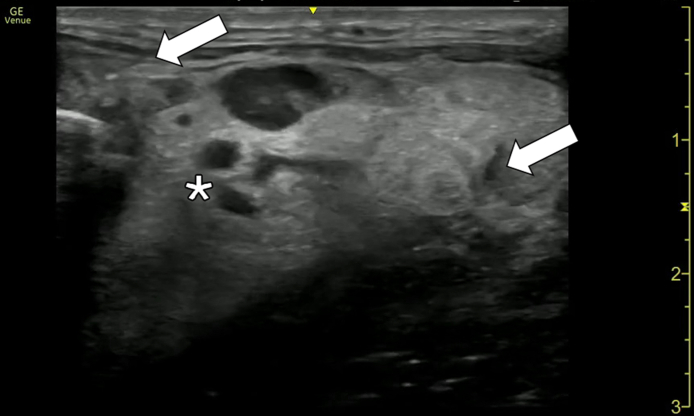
Transverse point-of-care ultrasound image of the submandibular neck revealing cobblestoning with subcutaneous fluid collection (arrows), lymphadenopathy, and loculated fluid collections along the left mandible (star).
